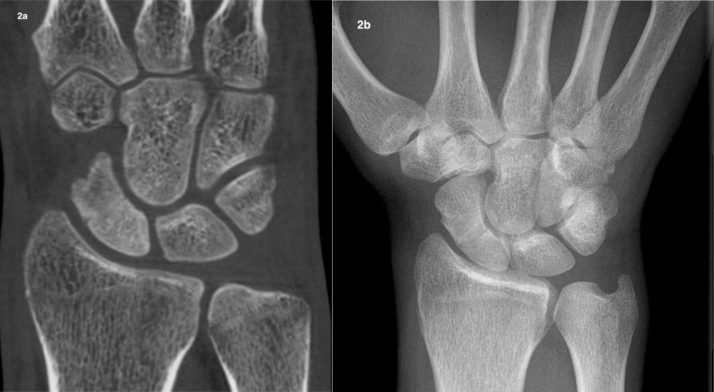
CBCT (a) of a 36-year-old, office worker with a right scaphoid fracture in Schoenberg’s zone III with complete healing according to all readers. XR (b) of the same patient shows no evidence of scaphoid healing.
